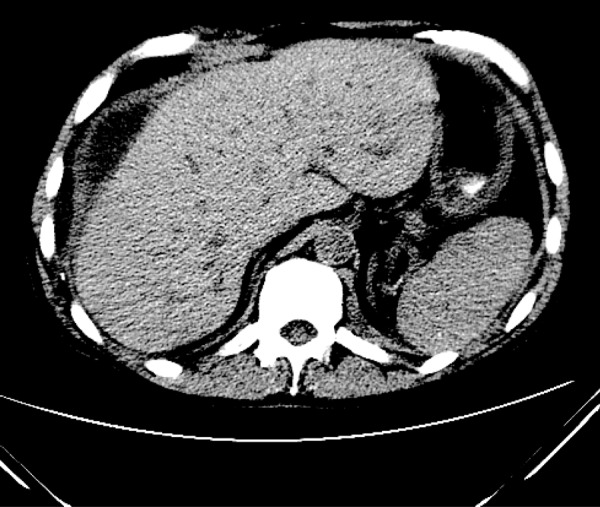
Axial, noncontrasted CT image slightly superior to the porta hepatis obtained at 8 months. The image demonstrates a persistent, encapsulated anterior perihepatic fluid collection (Culture negative). Although the collection has decreased in size from the previous exam, the “rind” is unchanged.
